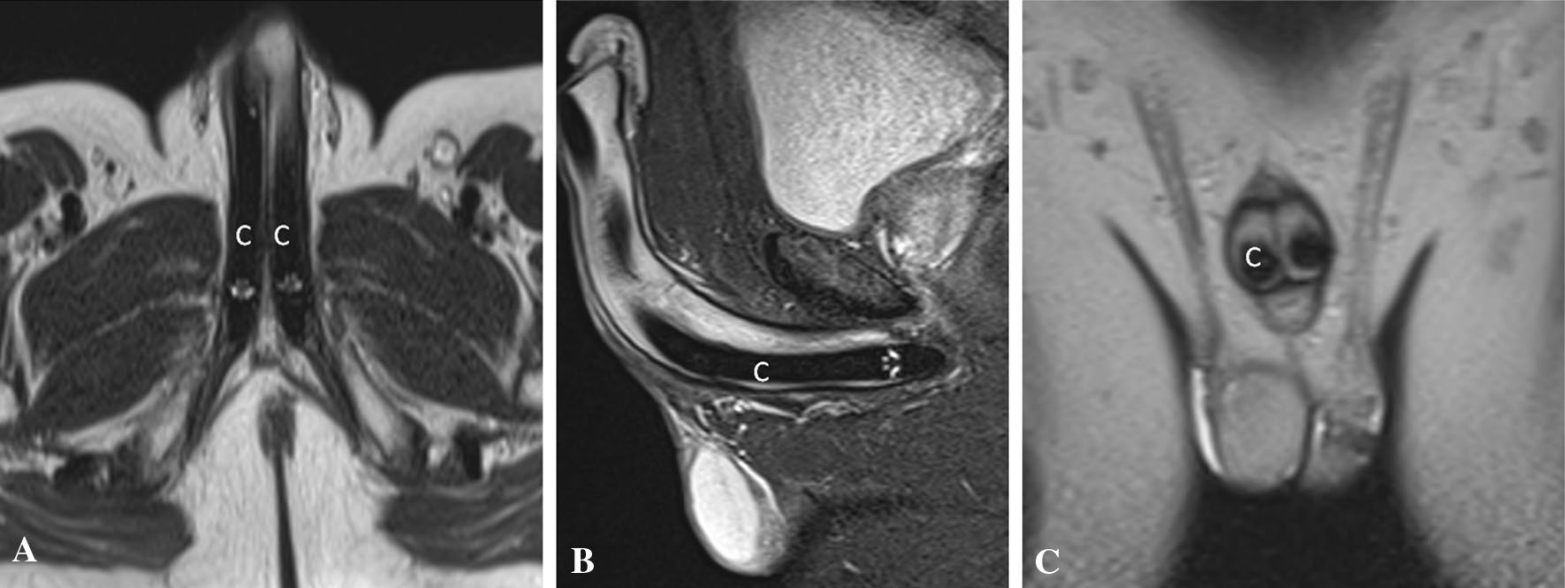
Appearance of normal malleable penile prosthesis in a 70-year-old male. Axial (a), sagittal (b), and coronal (c) T2-weighted MR images show paired T2 hypointense non-inflatable malleable penile prosthesis cylinders (c) in corpora cavernosaInflatable PP (IPP) can be of two types: (1) 3-pieces inflatable consisting of two inflatable cylinders placed inside corpora, a scrotal pump and a pelvic reservoir, (2) 2-piece inflatable containing two cylinders and a resipump (combined pump and reservoir) placed in the scrotum. All the components are interconnected by silicone tubing and are usually filled with normal saline [30] (Fig. 19).
